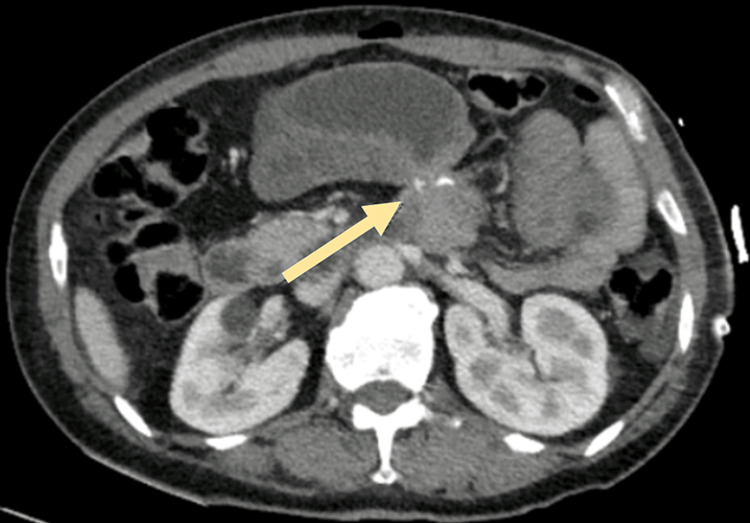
CT scan showing the abrupt stop sign in a proximal jejunal loop.
